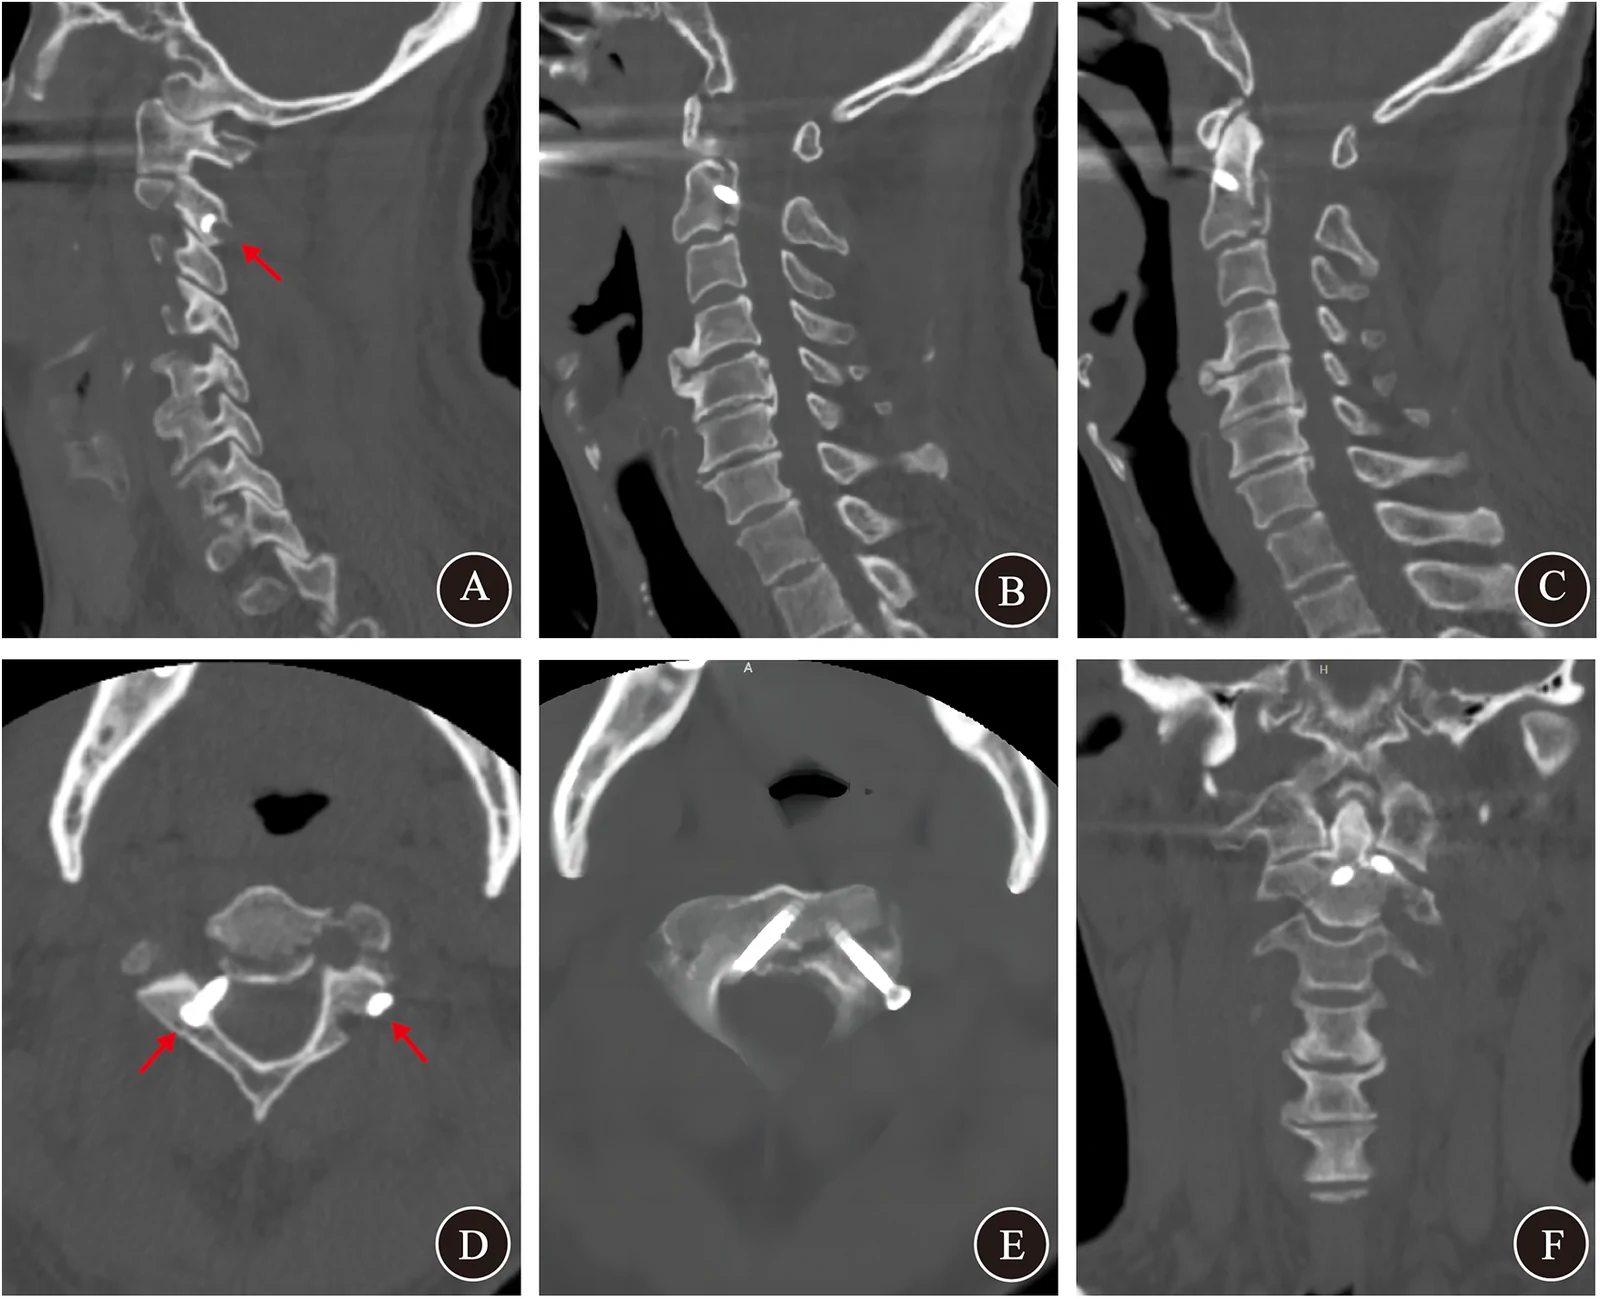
Postoperative cervical spine CT demonstrated accurate screw placement and satisfactory fracture reduction. (A–C) Sagittal views of the C2 cannulated screws, clearly showing the entry point (red arrow) and the trajectory of screw insertion. (D,E) Axial views of the C2 cannulated screws; panel D highlights the entry point (red arrow), while panel E demonstrates a significantly narrowed fracture gap compared to the preoperative status. (F) Coronal view of the C2 cannulated screws.
